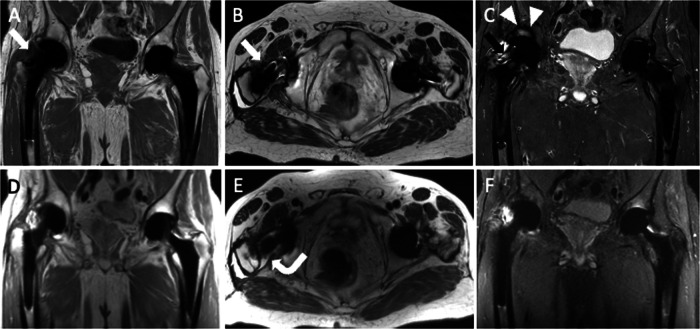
Pelvis MRI performed with MARS (A coronal T1-weighted; B axial T2-weighted; C coronal STIR) and MAVRIC (D coronal proton density-weighted; E axial T2-weighted; F coronal STIR) sequences. MAVRIC images are a bit blurred if compared with MARS, but reduce artifacts and signal loss improving imaging clarity close to metal components. Note the signal loss (arrows) near the prosthetic neck (A, B, C) and poor fat suppression in the acetabulum (arrowheads, C) on MARS images. Also, MAVRIC is more effective in depicting the right THA effusion (curved arrow, E)
